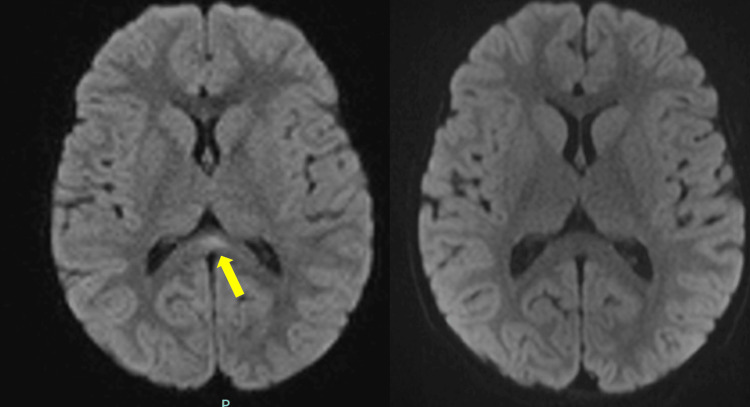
Case 1: Axial DWI of the brain (A) Initial MRI (March 2021): focal diffusion-restricted hyperintensity involving the splenium of the corpus callosum. (B) Follow-up MRI (June 2021): complete resolution of the previously described splenial diffusion restriction. DWI, diffusion-weighted imaging
